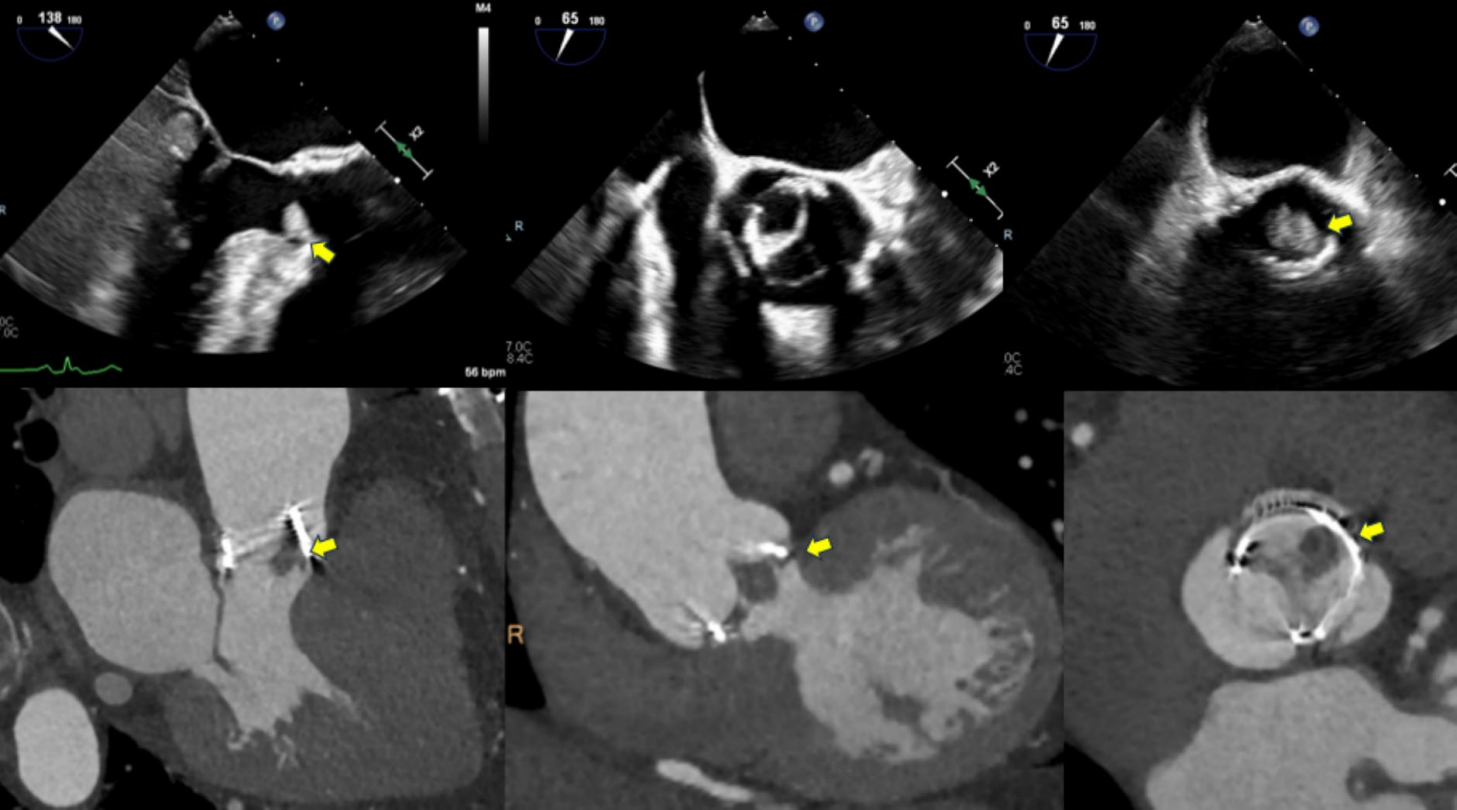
70-year-old female with prior surgical aortic valve replacement, presenting with acute limb ischemia and *Pseudomonas Aeruginosa* bacteremia, raising concern for possible infective endocarditis. Figure 1: *top panel*: transesophageal echocardiogram images with an echodensity on the aortic valve (arrow) suspicious for a vegetation, though the assessment was limited by artifacts. The *bottom panel*: gated cardiac CT imaging, clearly visualizing the vegetation (arrow). CT ruled out aortic root abscess or paravalvular infection. The patient was treated with appropriate antibiotics for infective endocarditis. This case underscores the utility of CT in clarifying equivocal echocardiographic findings in endocarditis management
